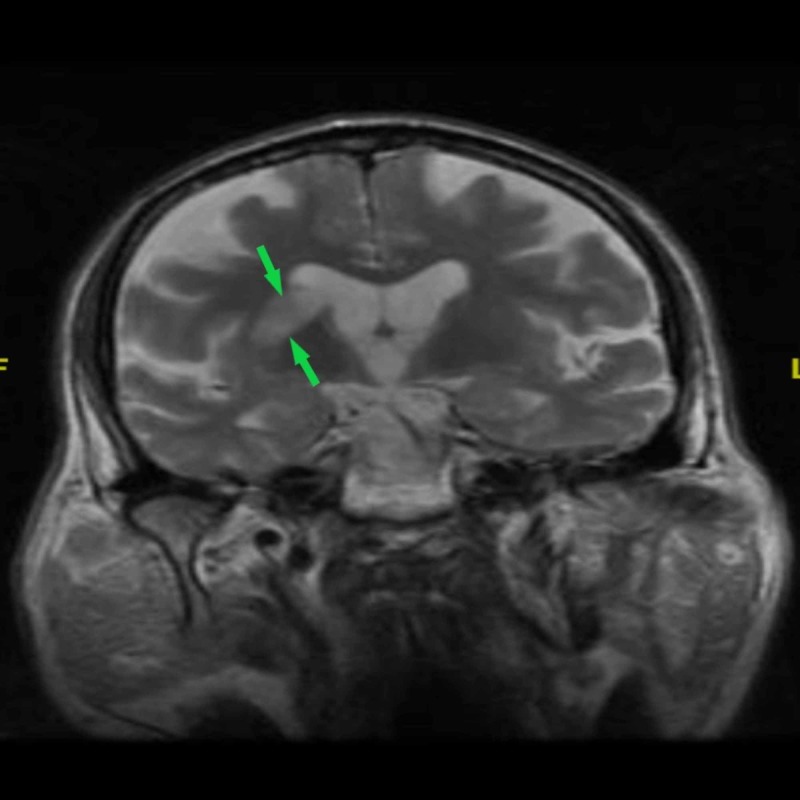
Brain MRI showing right corona radiata and right basal ganglia ischemic lesion (green arrows) in T2-weighted MRI (coronal plane) MRI: magnetic resonance imaging
